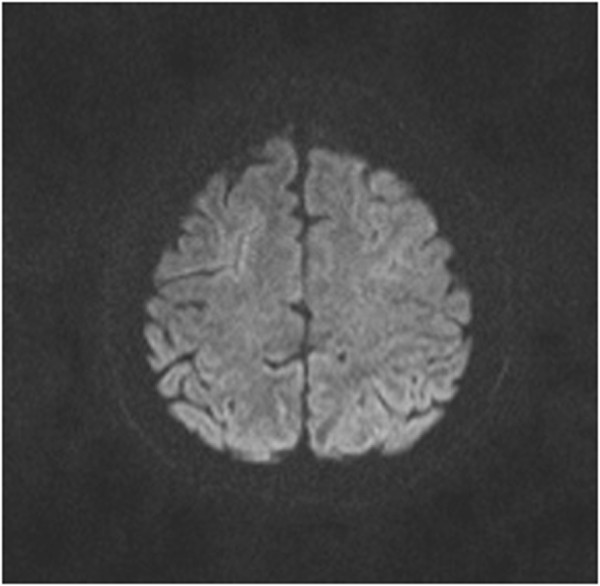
Diffusion-weighted axial magnetic resonance imaging brain image showing no abnormalities.
